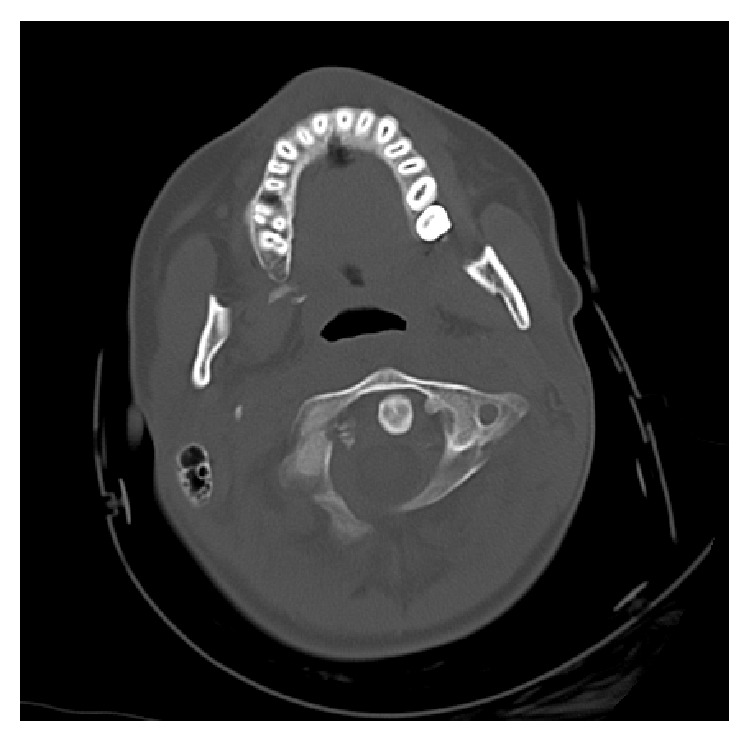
Axial CT reconstruction image shows avulsion fracture of the right occipital condyle (type III according to Anderson-Montesano classification).
